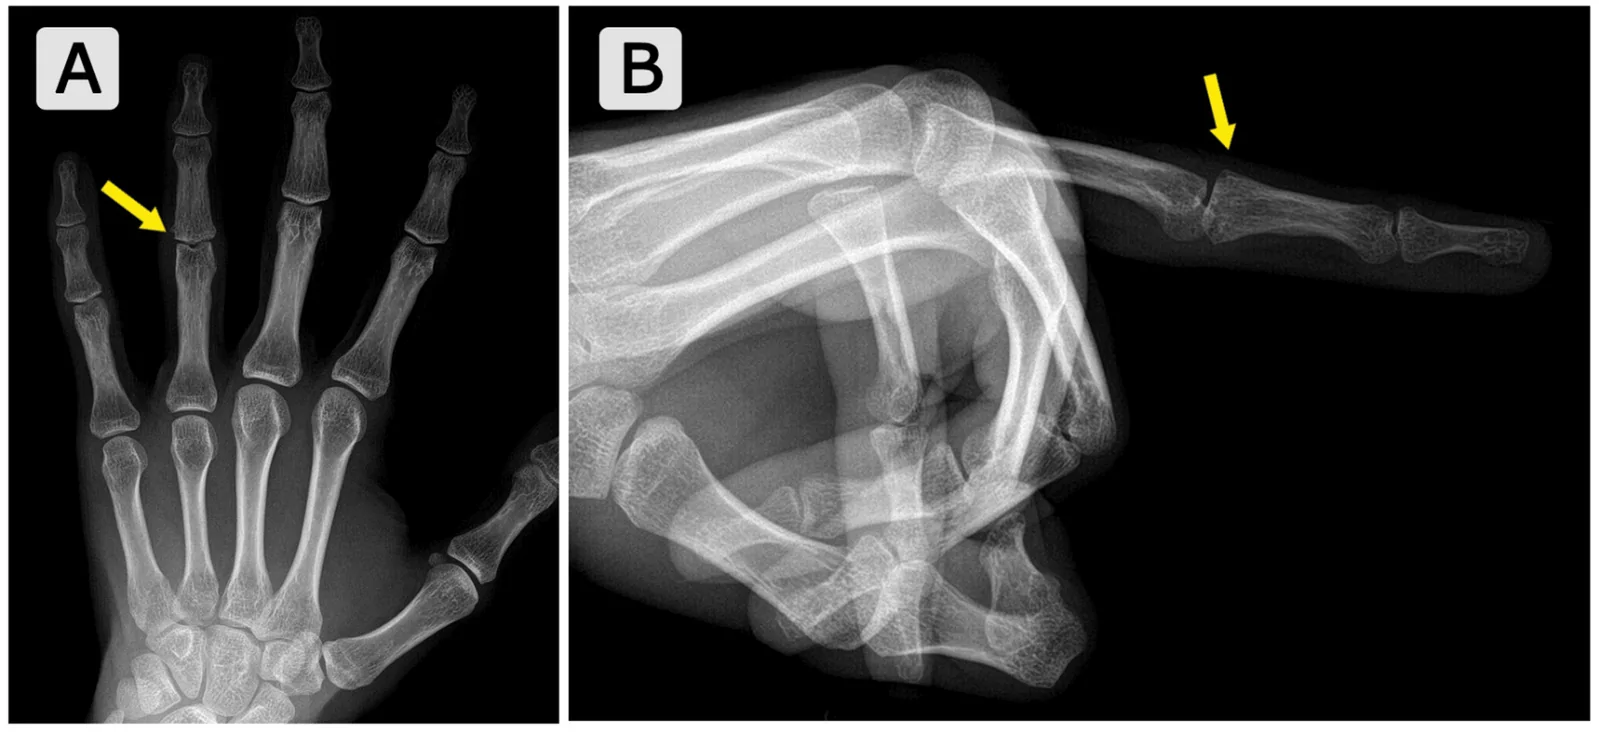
Two-year follow-up radiographs. (A) AP view demonstrating maintained reduction, preserved joint congruity, complete fracture union, and no radiographic evidence of post-traumatic osteoarthritis (arrow). (B) Lateral view confirming full active PIP extension (0°) with no extension lag and maintained joint alignment (arrow) AP: anteroposterior, PIP: proximal interphalangeal
